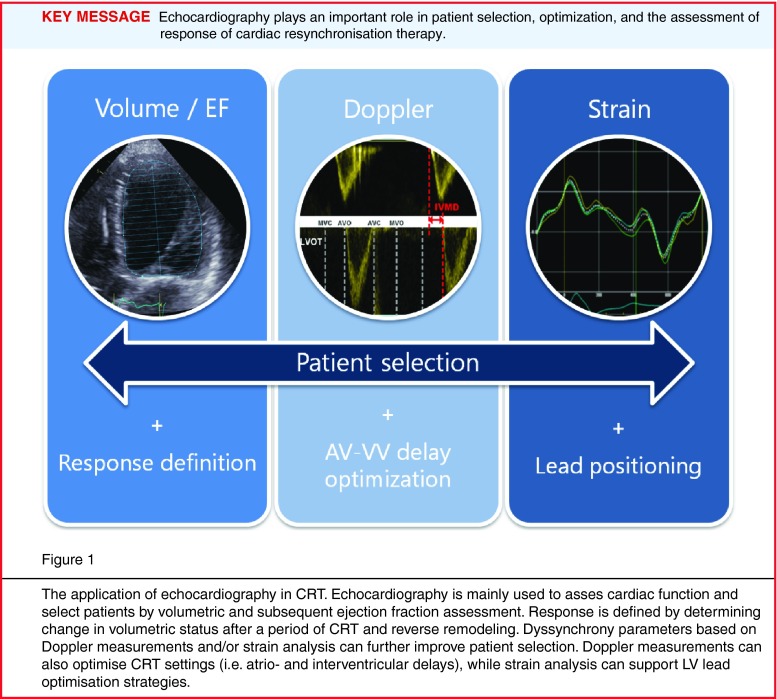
The application of echocardiography in CRT. Echocardiography is mainly used to asses cardiac function and select patients by volumetric and subsequent ejection fraction assessment. Response is defined by determining change in volumetric status after a period of CRT and reverse remodeling. Dyssynchrony parameters based on Doppler measurements and/or strain analysis can further improve patient selection. Doppler measurements can also optimise CRT settings (i.e. atrio- and interventricular delays), while strain analysis can support LV lead optimisation strategies
